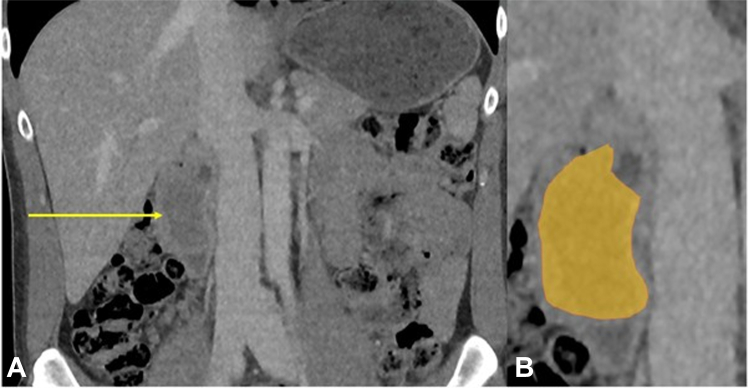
A, Coronal portal venous phase CT showing a low-density filling defect in the duodenum (*arrow*). B, Magnified view showing the lesion in *yellow color*.
